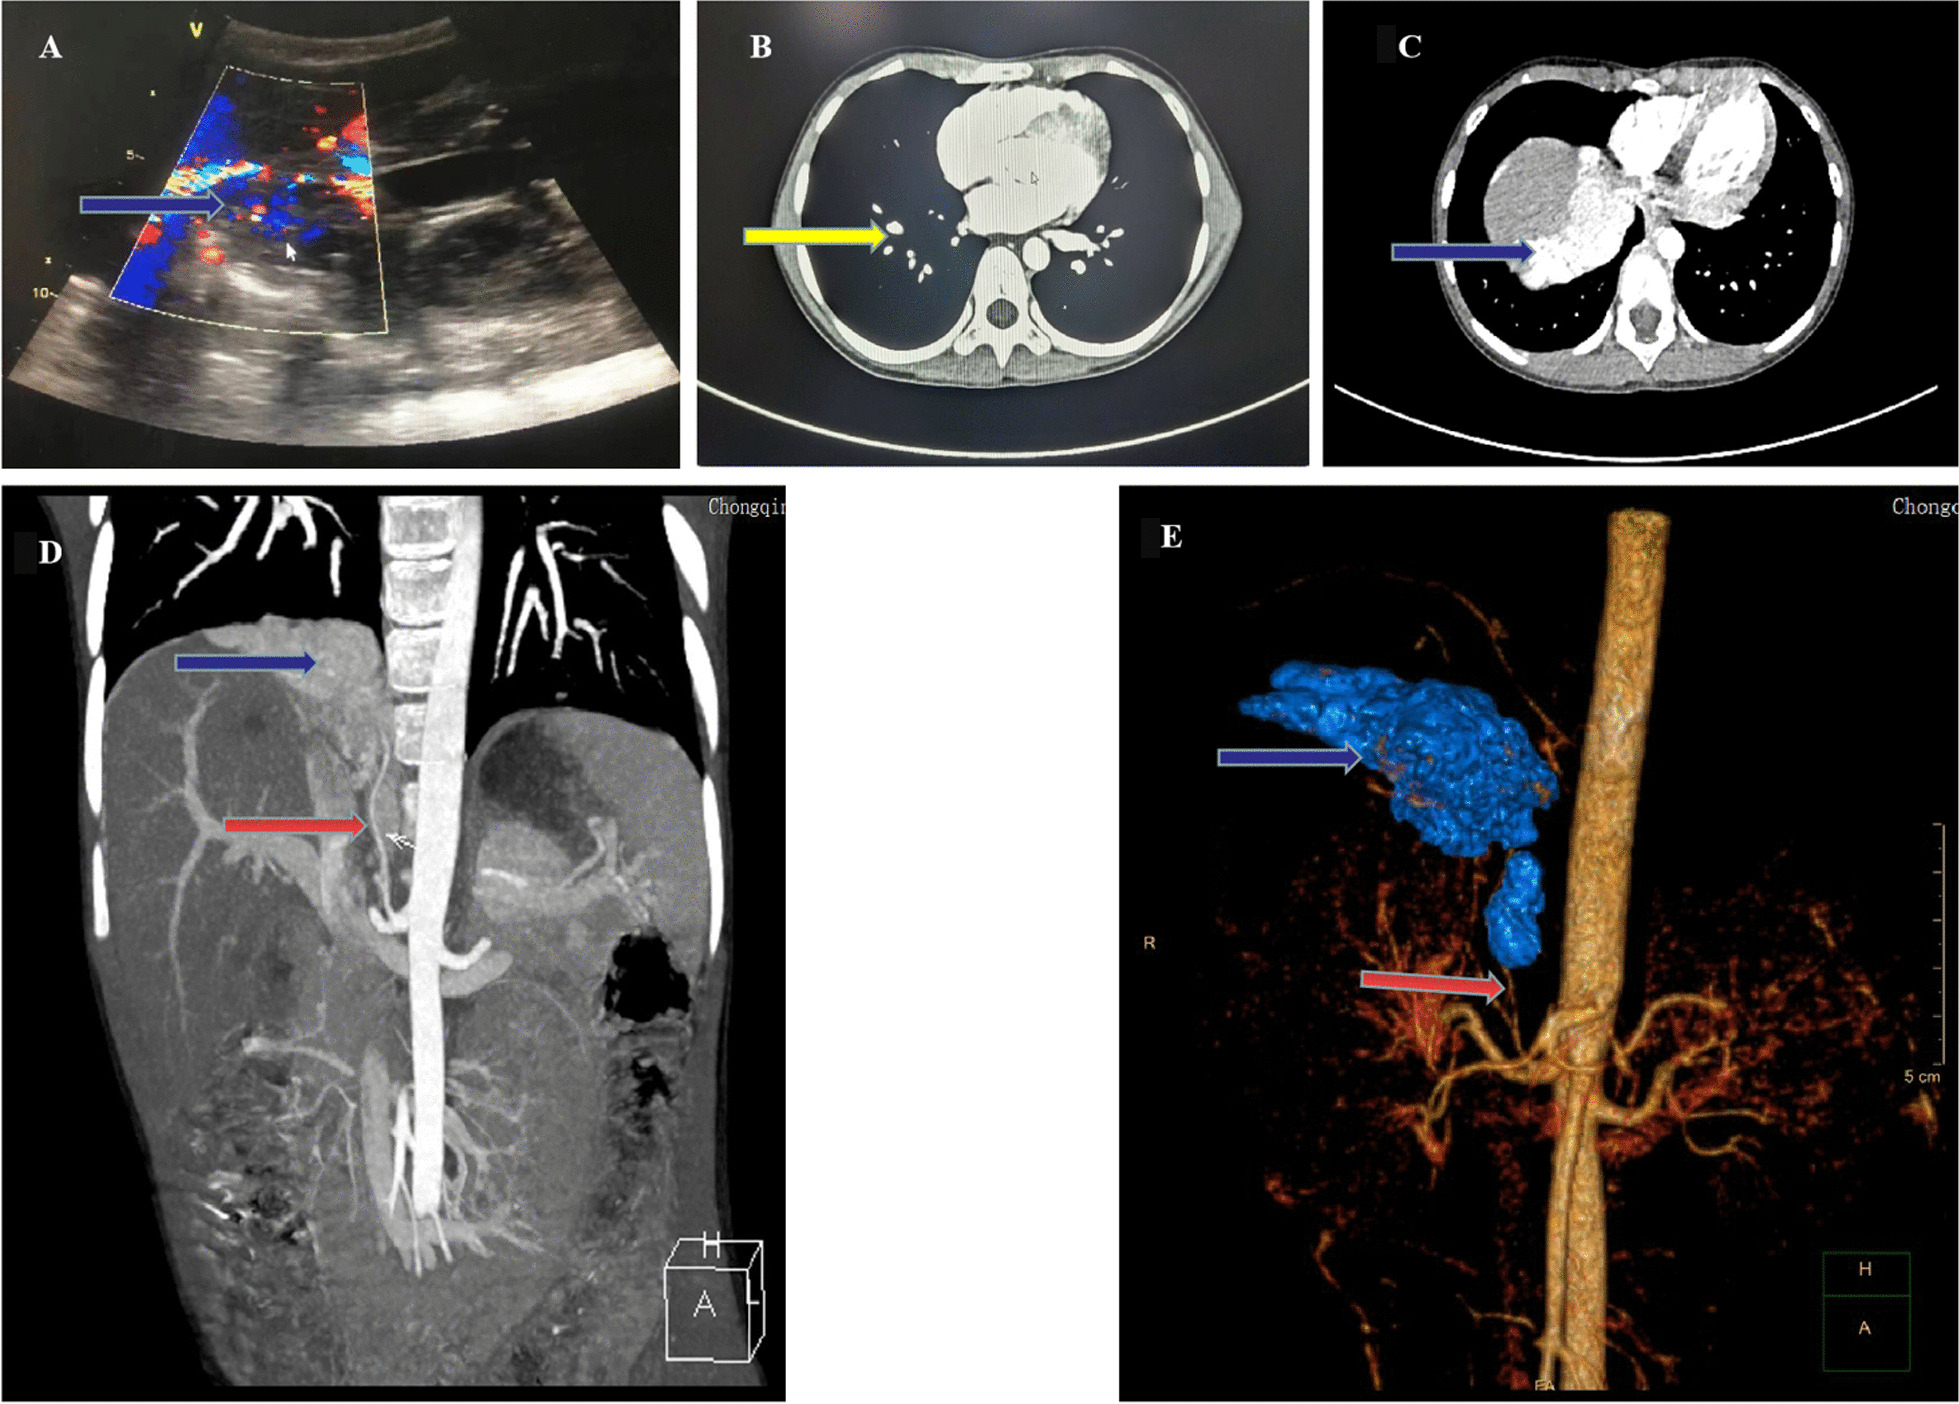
Abdominal ultrasound result showing that exploration and size of the right adrenal area was about 1.1 cm × 1 cm hypoechoic structure, with rich internal blood flow signal (blue arrow in A). Chest CT results showed many nodules in both lungs, and the largest nodule (yellow arrow in B) was located in the middle lobe of the right lung, with a size of about 8.2 mm × 7.0 mm. Abdominal CT results showed that the right adrenal gland was not clearly displayed and multiple enhanced nodules were found inside, with the largest having a size of 10.8 mm × 17.2 mm × 17.8 mm (blue arrow in C). CT angiography showed that the tumor lesion (blue arrows) was compressed adjacent to the inferior vena cava, and the right inferior adrenal artery had vascular branches (red arrows) into the tumor lesion (D and E)
